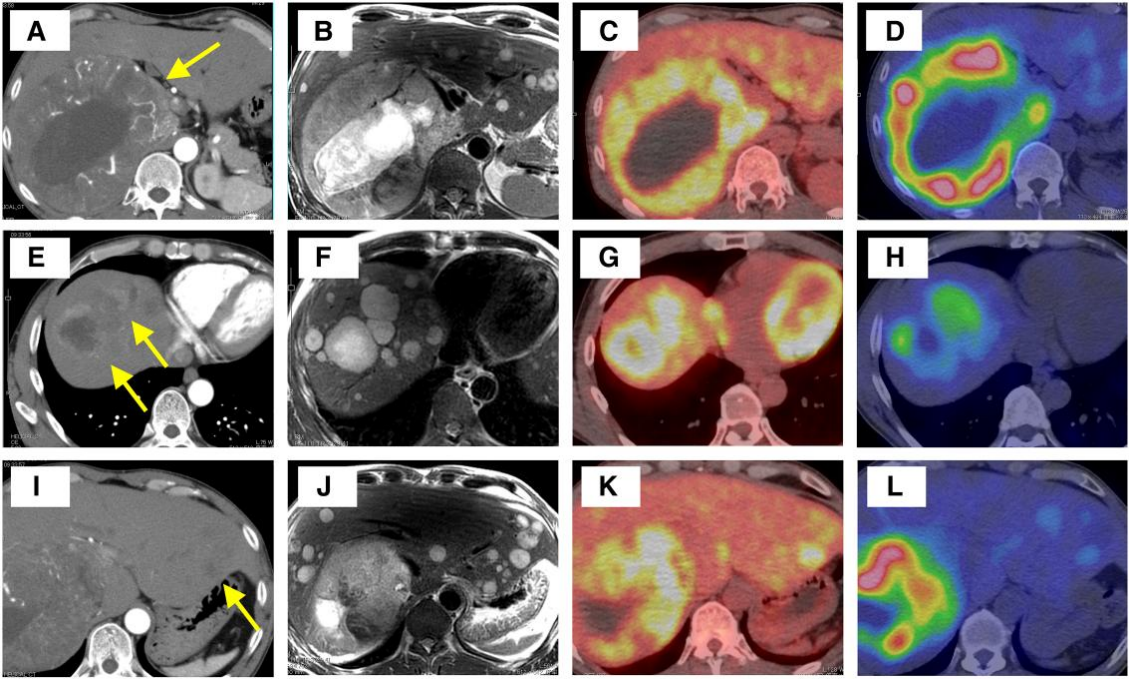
Imaging of adrenal and liver nodules. (A-D) Adrenal mass. (E-H) Liver nodule in the right lobe (S7, S8). (I-L) Liver nodules in the left segment (S2, S3). (A, E, I) Contrast CT. (B, F, J) MRI. (C, G, K) ^123^I-MIBG scintigraphy. (D, H, L) FDG-PET. Abbreviations: CT, computed tomography; FDG-PET, fluorodeoxyglucose positron emission tomography scan; MRI, magnetic resonance imaging; MIBG, metaiodobenzylguanidine.
